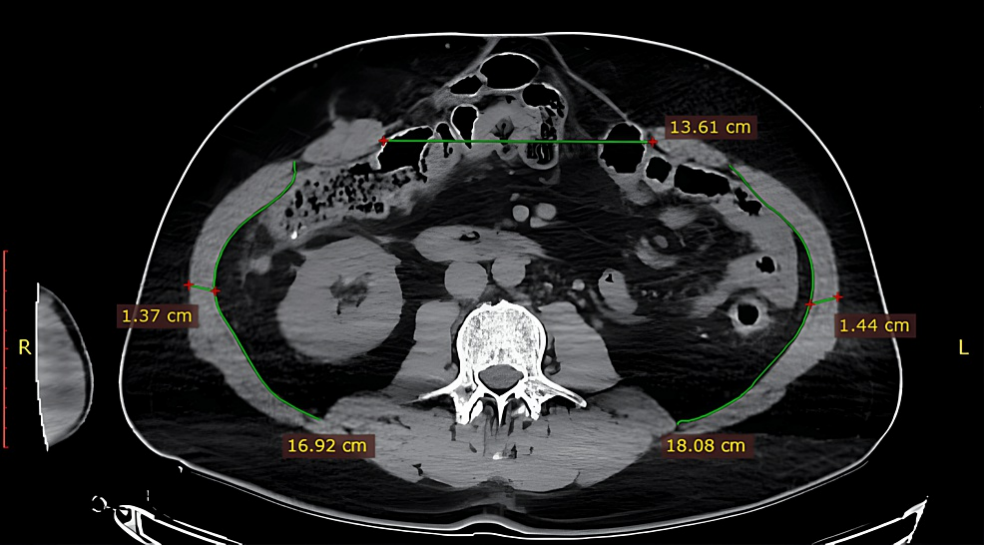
Preoperative computed tomography scan A simple computed tomography scan was conducted to determine information related to the hernia defects’ size, dimensions, and precise location
